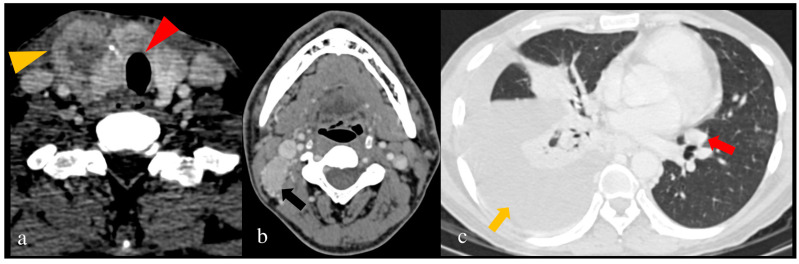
Stage II papillary carcinoma thyroid in a 40-year-old male patient shows: (a) extrathyroidal extension (yellow arrowhead) and tracheal cartilage involvement without tracheal mucosal involvement (SHIN II (red arrowhead)) (b) metastatic right level II node (black arrow), (c) metastatic right pleural effusion (yellow arrow), and metastatic left lung lower lobe nodule (red arrow) on contrast-enhanced computed tomography (CECT).
